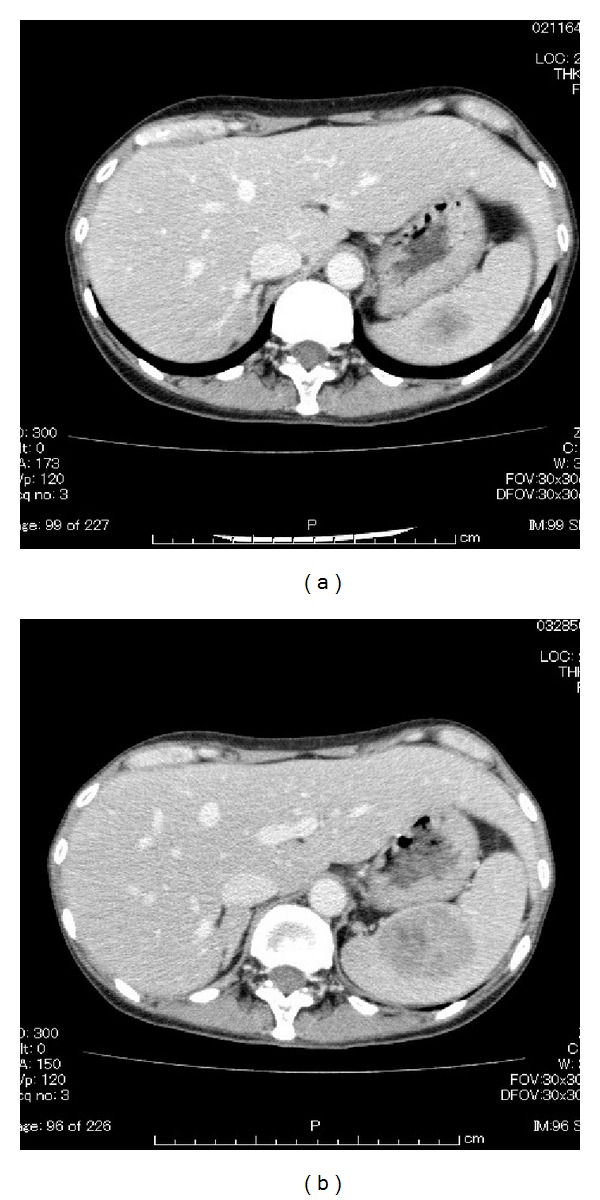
(a) CT showing probable spleen metastasis. (b) The mass found in the spleen enlarged.
